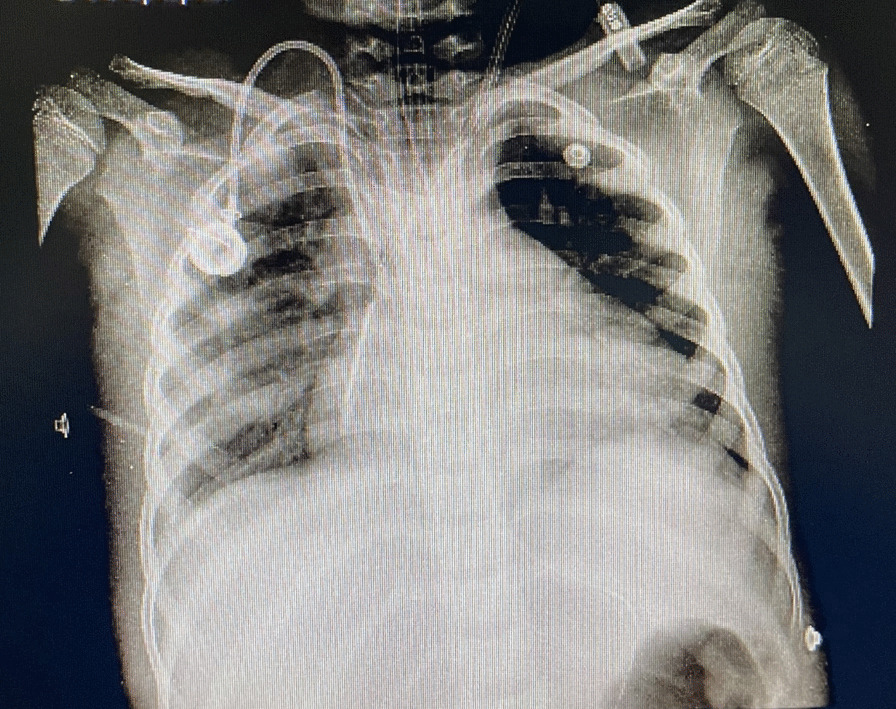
A chest radiograph showing an opaque patch in the peripheral right hemithorax of the lungs
